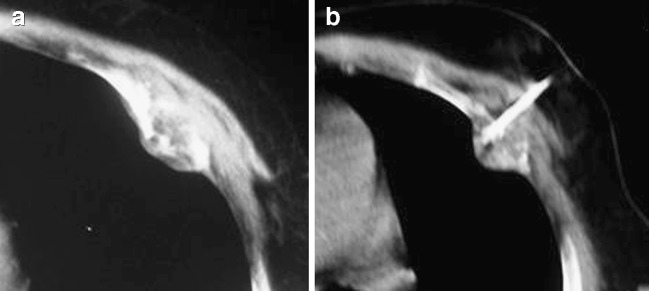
Computed tomography scan image during the electrode placement inside a a metastatic lesion involving a rib (the primary neoplasm originates in the lung). Immediately after the radiofrequency session the contrast enhancement CT scan revealed a hypodense area (necrosis) inside the lesion. Before the radiofrequency session, the average pain score was 6. During the first 24 h after RF, it was 4, and 1, 4 and 8 weeks later the average pain scores were 2, 2 and 1 respectively
